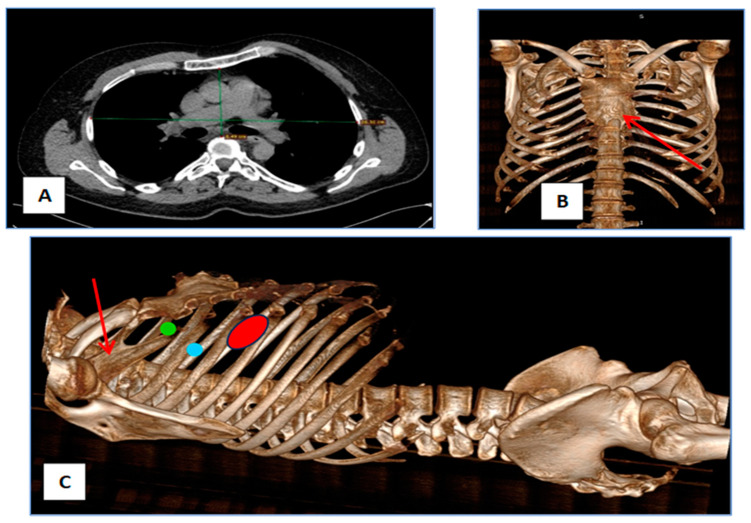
(A) CT scan demonstrating pectus excavatum with a Haller index of 3.1. (B) CT scan and 3D bone reconstruction: the red arrow denotes a malformation of the upper sternum. (C) Lateral view: the red arrow highlights the fusion of the first and second ribs. The red dot marks the incision site in the fourth intercostal space, the green dot indicates the position of the 3D camera, and the blue dot marks the location of the Chitwood clamp.
